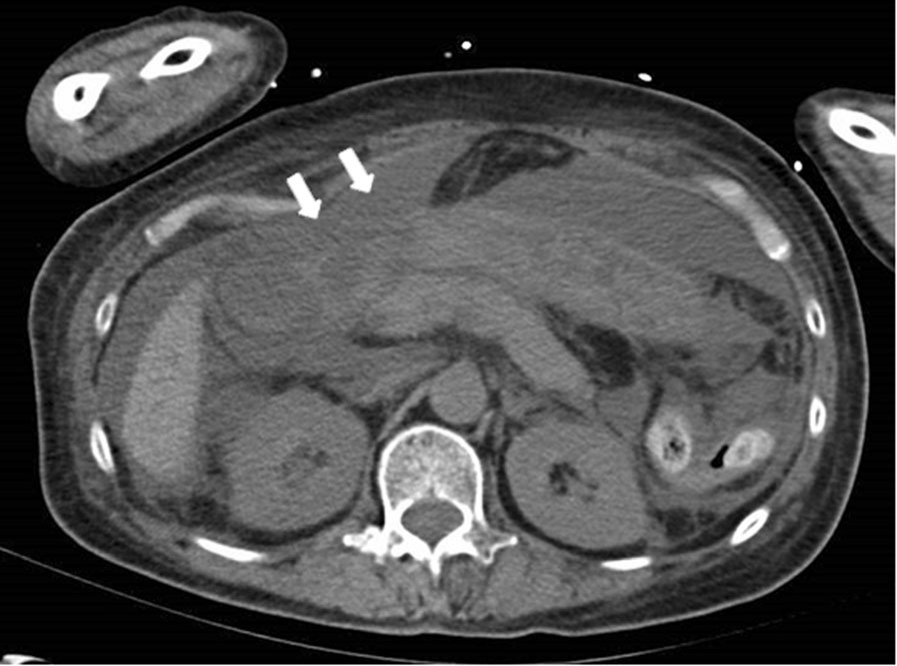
Abdominal CT. The CT scan reveals massive fluid accumulation and an irregular duodenal wall
